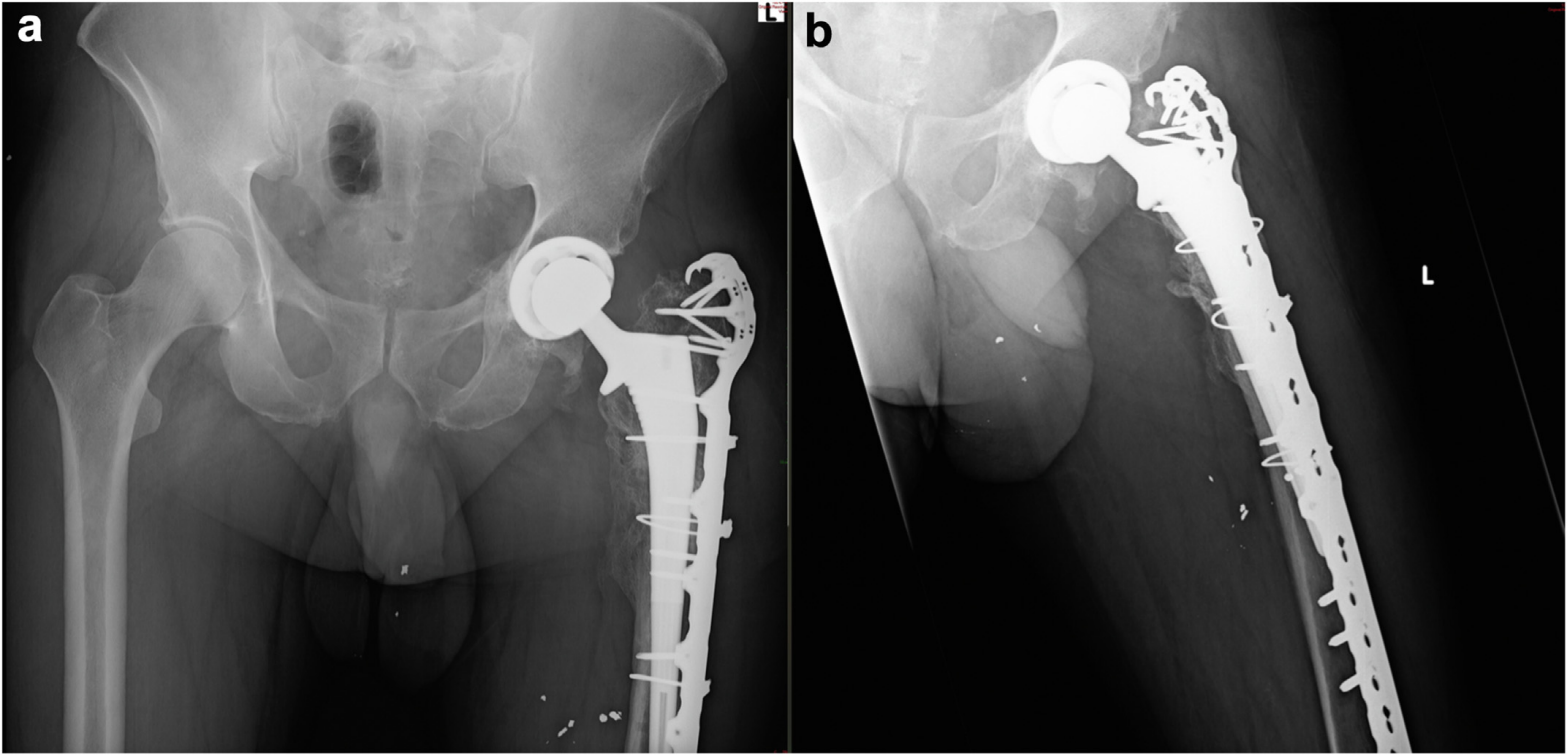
(a) AP and (b) axial X-ray views of the left proximal femur and hip joint at the 7-month follow-up, showing complete fracture healing and proper orientation of the THA components. AP, anteroposterior; THA, total hip arthroplasty.
